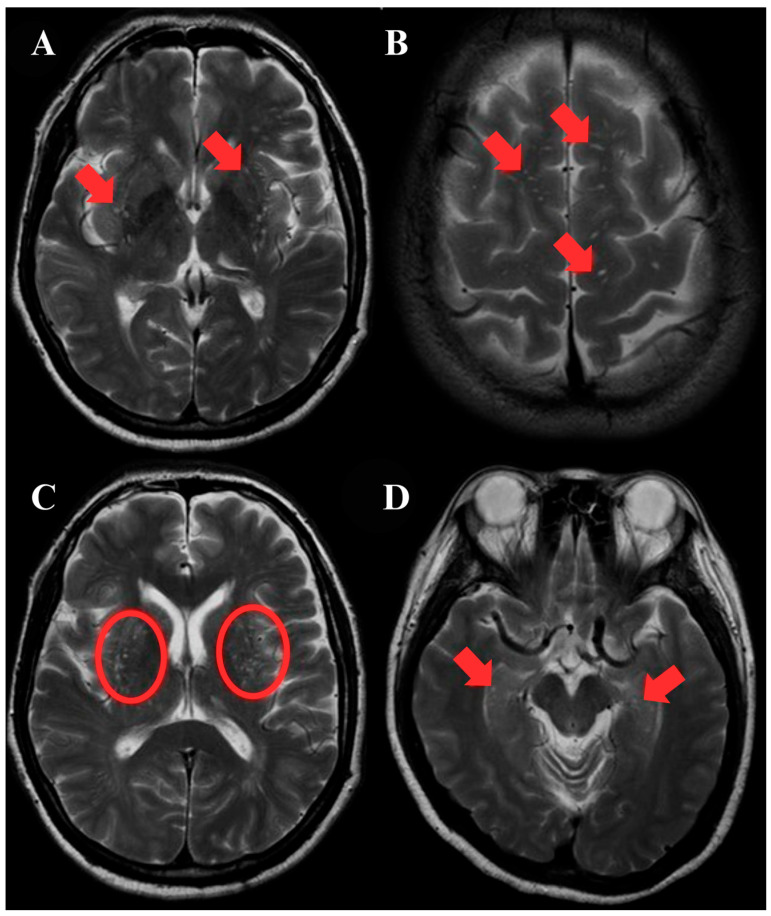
Structural T2-weighted images indicating the spatial distribution of enlarged perivascular spaces (ePVS) in subjects with ePVS. Images highlighting location (arrows indicate ePVS presence in different brain regions). Panels (A,C) refer to subjects showing more than one ePVS located exclusively in the basal ganglia at the globus pallidus, caudate nucleus, and putamen (red arrows in panel (A) and red circles in panel (C)). Panel (B) shows those subjects with multiple ePVS distributed in bi-hemispheric subcortical white matter, such as within the centrum semiovale. Panel (D) shows subjects with multiple ePVS at the bilateral hippocampus.
